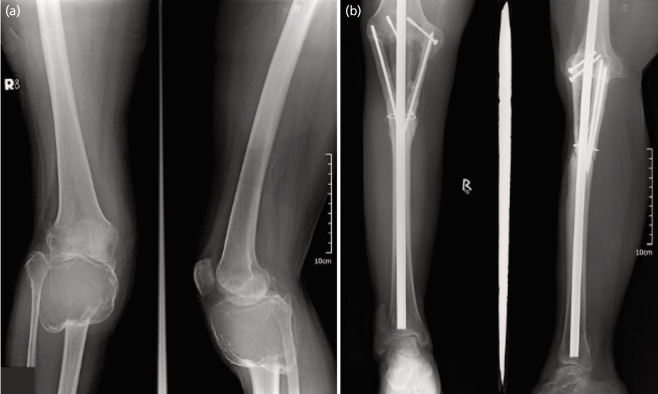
KRA with IM nail in a 51/F. (a) Pre-operative radiographs show a giant cell tumour of the proximal tibia, for which proximal tibia resection and KRA was performed. (b) Three years post-operatively show good union with fibular grafts proximally and distally.
